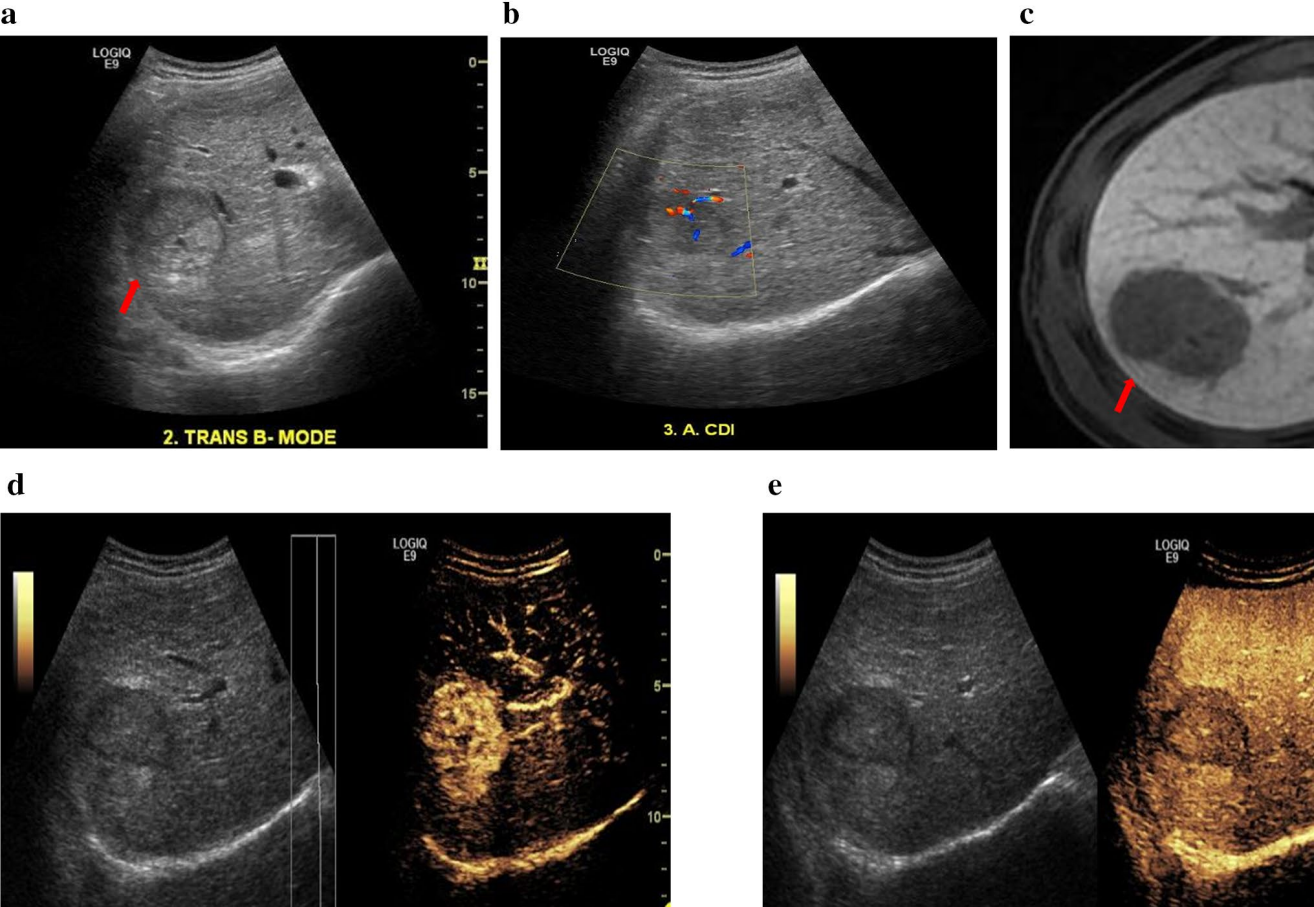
51-year-old male patient with hepatocellular carcinoma (HCC). a Pre-contrast harmonic B-mode shows isoechoic HCC. b In Colour Doppler imaging HCC shows some peripheral and central vascularity. c CE-MRI used as the reference diagnosis, indicating the HCC in the hepatobiliary phase. d Arterial phase post-Sonazoid injection shows homogeneous hyper-enhancement. e Kupffer phase at approximately 10 min shows washout with well-defined lesion margins, hypo-enhancement and strong contrast retention in the liver parenchyma
